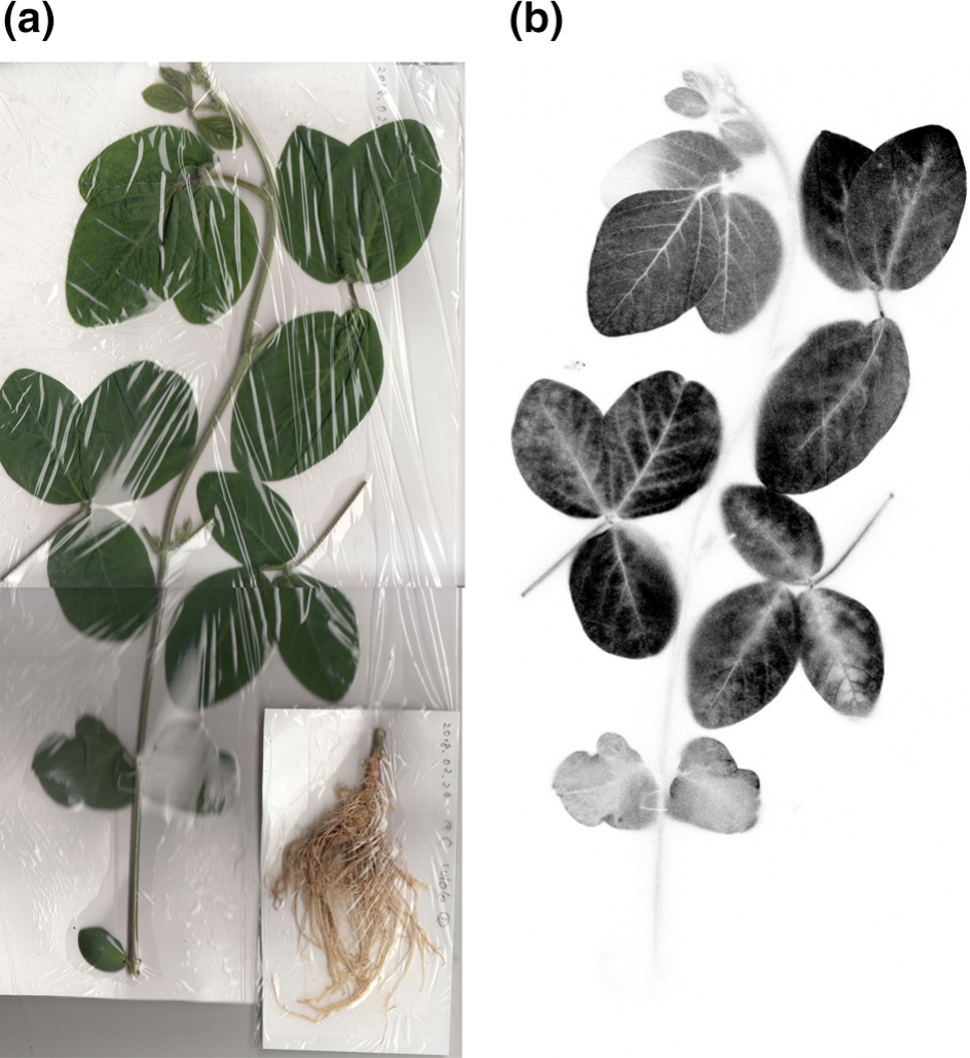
Photosynthate image in a plant. The ^14^CO_2_ gas was produced and exposed to a soybean plant for 30 min. Then, the plant was exposed to an imaging plate (IP) for 30 min. a A picture of the plant. b The radiograph of the image in the IP. (Color figure online)
